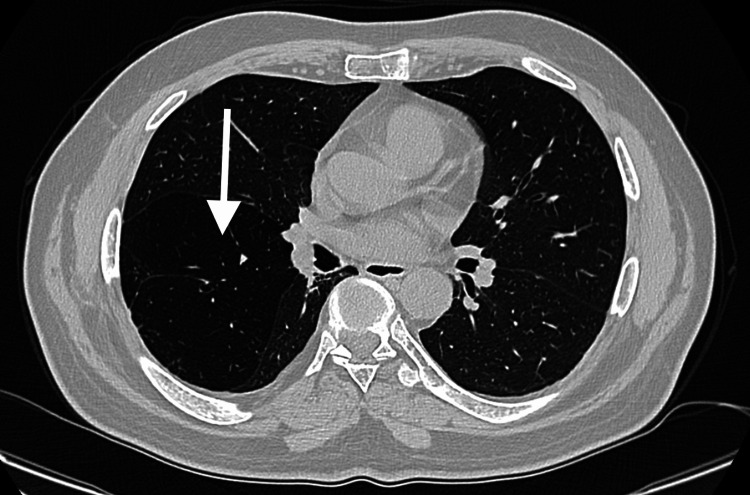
Focal hyperlucency in the right upper lobe Axial chest CT image in lung window showing focal hyperlucency and overdistension involving the posterior segment of the right upper lobe (white arrow).
